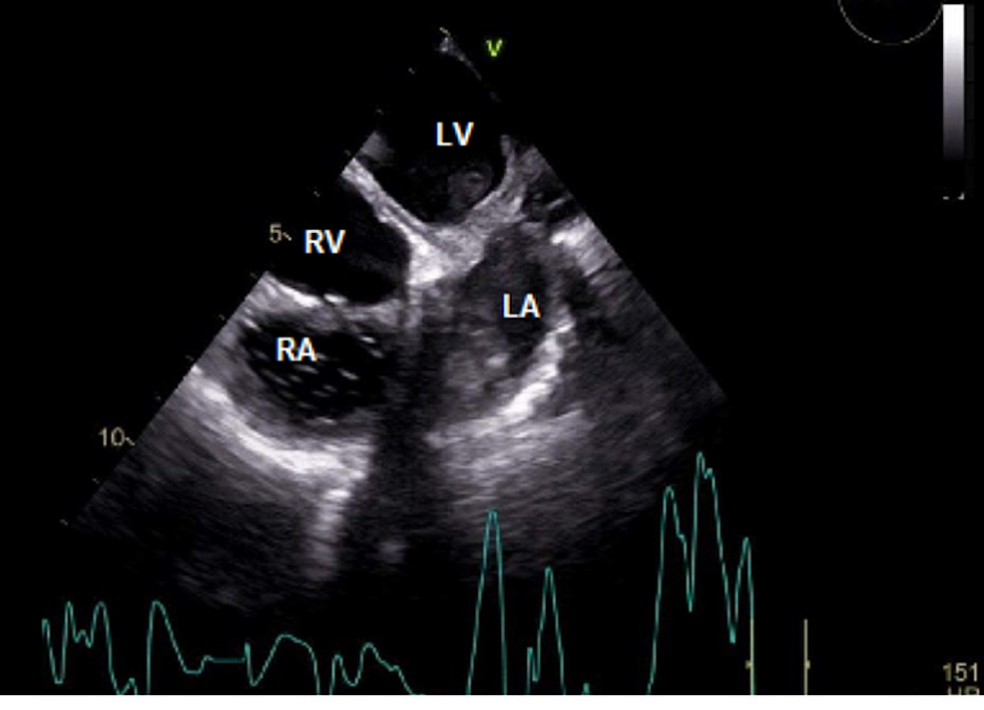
Transesophageal echocardiogram demonstrating left atrial mural thrombus. RA: Right atrium; LA: Left atrium; RV: Right ventricle; LV: Left ventricle
